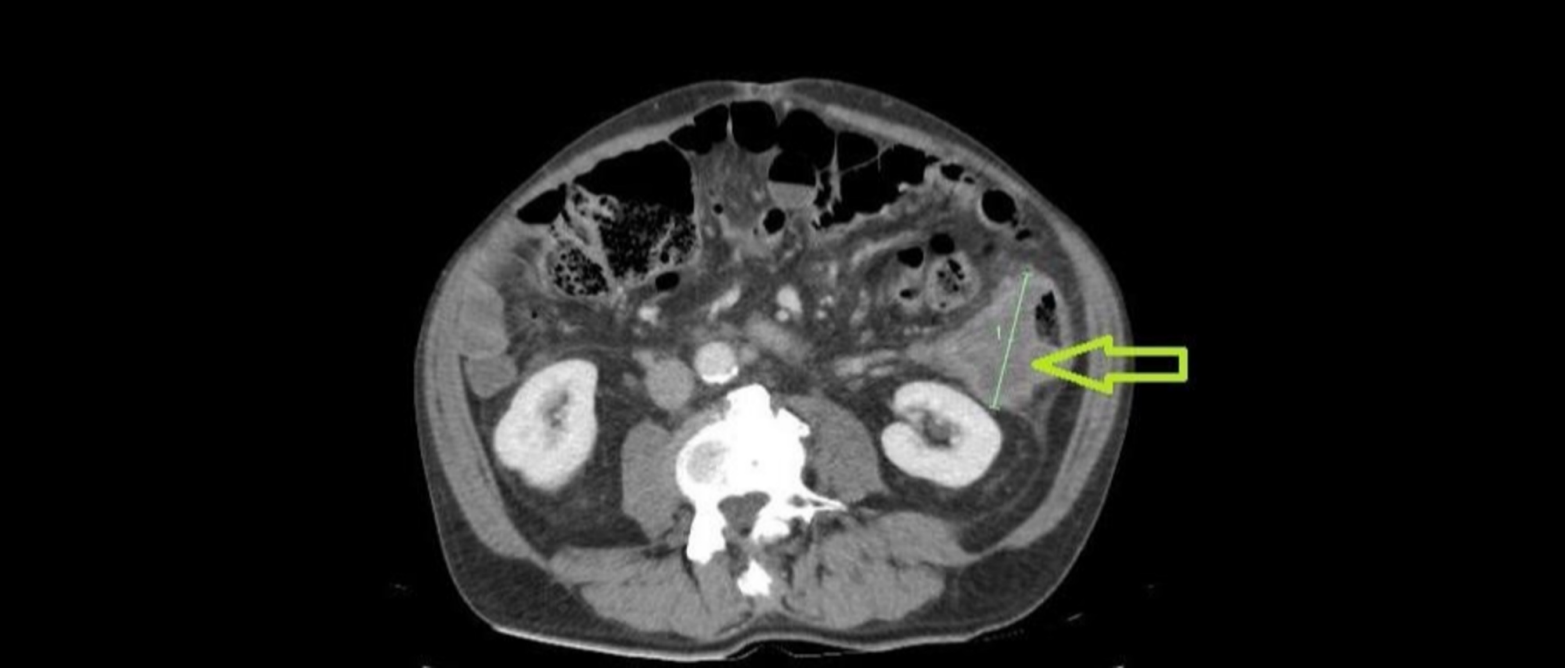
Abdominal CT scan post-contrast showing a large exophytic solid mass arising from the mid-descending colon, measuring approximately 7 cm x 6.5 cm x 6 cm.
